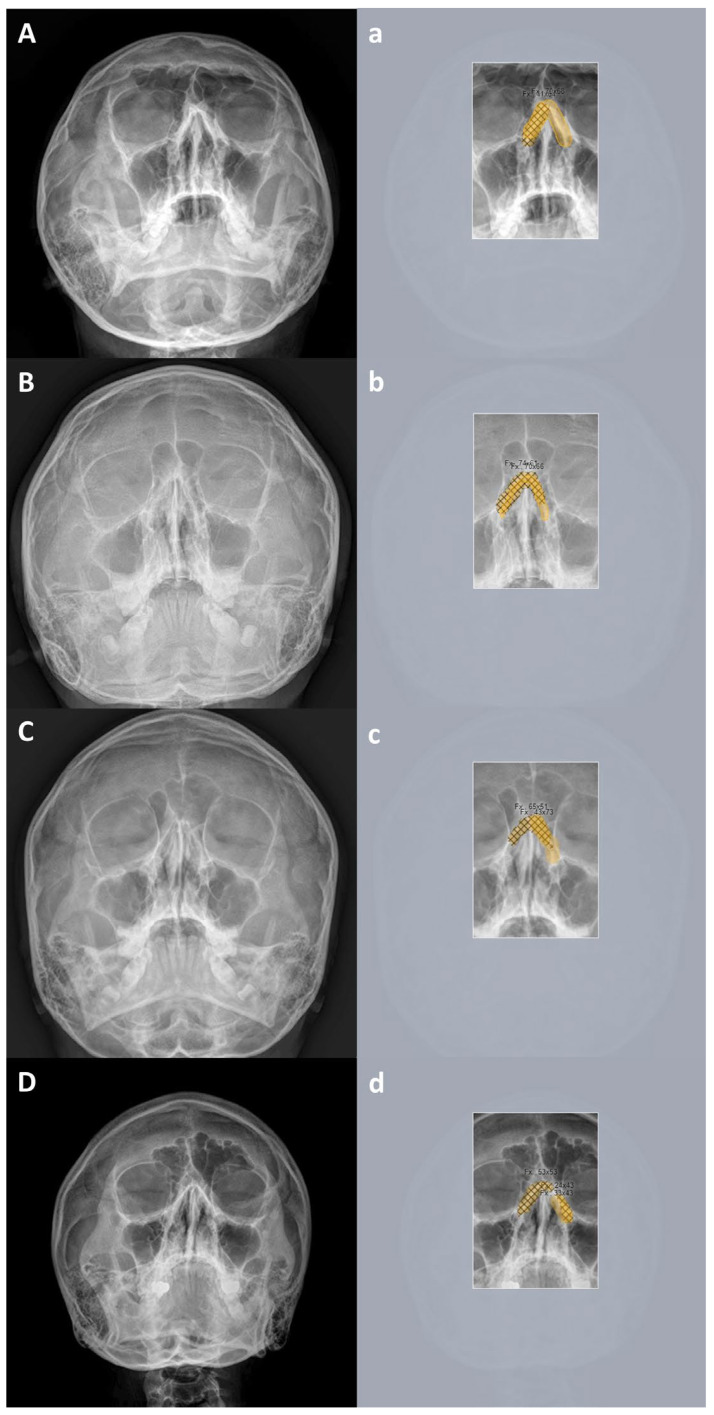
Representative cases of incorrectly predicted classes in the test dataset using the no-code platform with a segmentation labeling method. (Left) Radiographic images of the Skull Waters’ view with the removal of right/left markings from the corners of the X-ray. (Right) The orange color represents the area labeled by the authors using a segmentation method, while the hatched part corresponds to the pixel area predicted as fractures by the deep-learning model. The images (A,a), (B,b), (C,c) and (D,d) show normal nasal radiographs misclassified as fractures.
